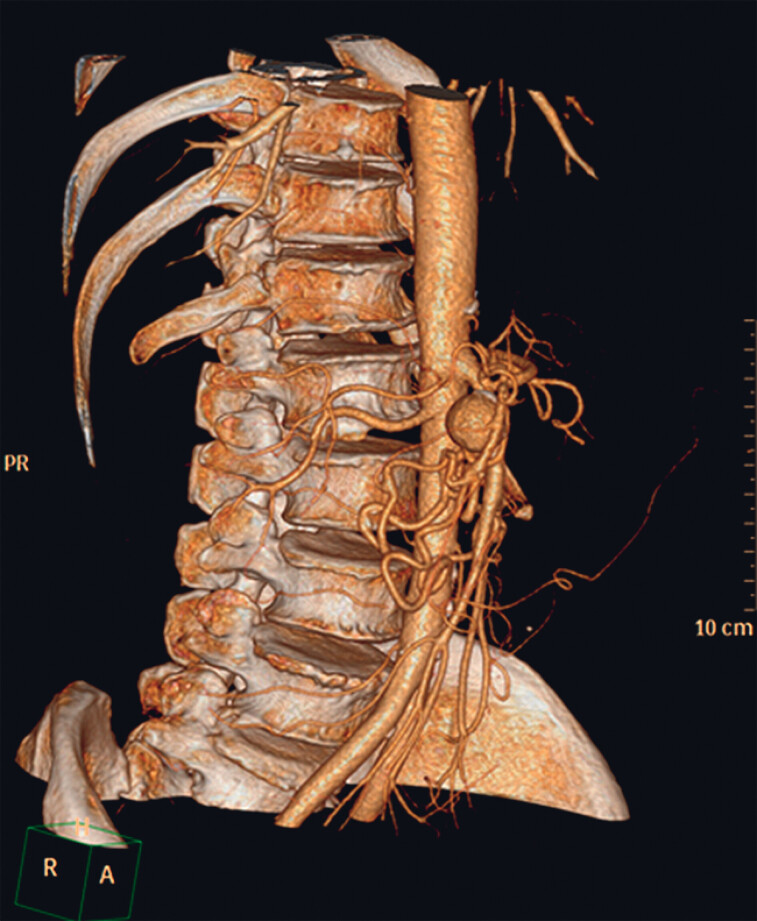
Gastroduodenal artery pseudoaneurysm (GDAP) shown by gastric vascular CT angiography (CTA).
